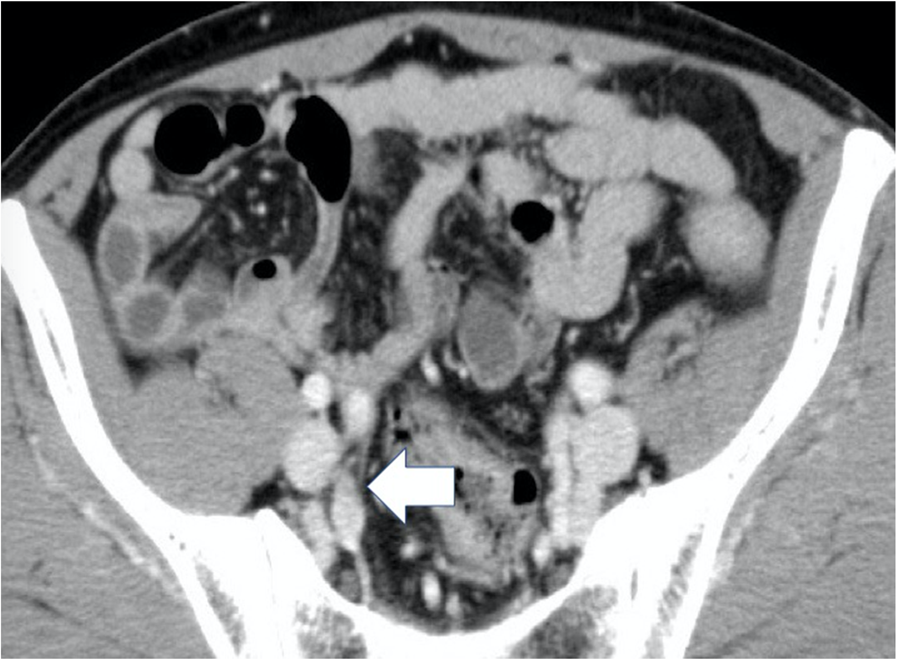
Contrast-enhanced computed tomography performed prior to initial surgery. The image shows an unusual area of soft tissue around the right internal iliac artery (arrow)
